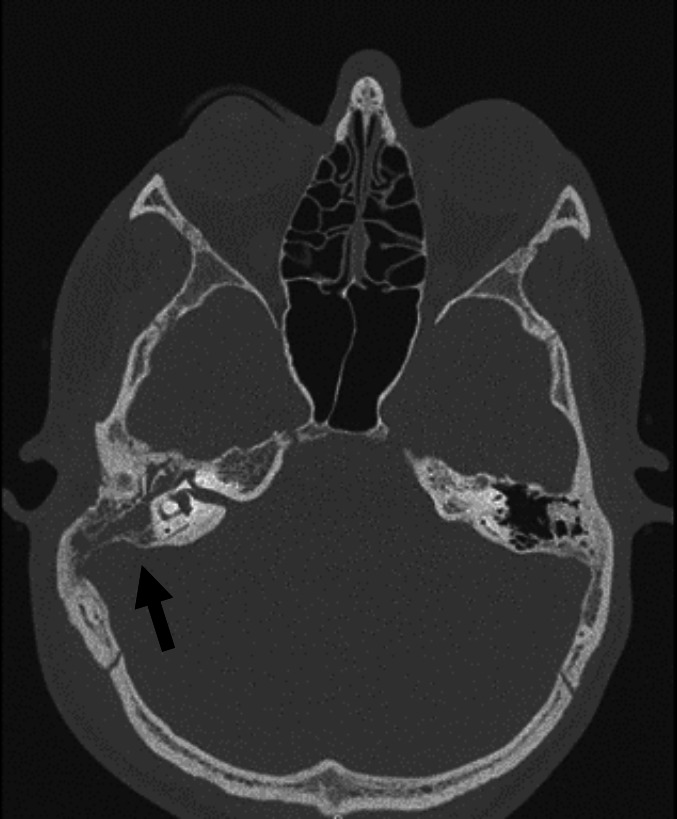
Computed tomography showing obliteration of the mastoid and tympanic cavity with ossicular erosion compatible with otomastoiditis.
